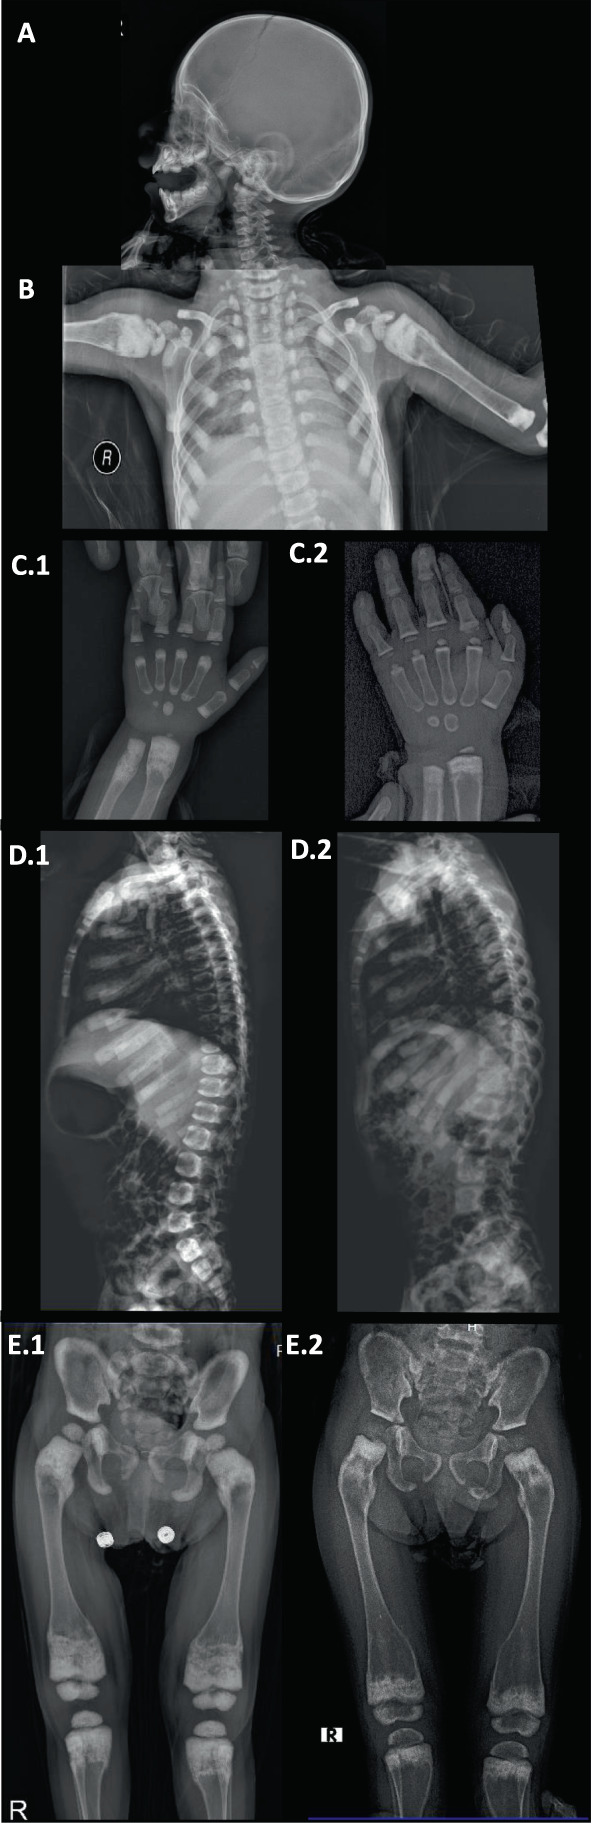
Patient 4. Radiographic findings in early childhood. (*A*) At age 2 years, the skull has slightly increased vertical diameter. (*B*) There is marked metadiaphyseal osteosclerosis and widening of the proximal humeri, marked osteosclerosis and some widening of the ribs, and osteosclerosis of the spine, clavicles, and scapulae. (*C.1*,*C.2*) Between ages 2‐1/3 and 4‐1/3 years, osteosclerosis has decreased in the metaphyses and carpals in the hands and wrists, and metadiaphyses in the radius and ulna. Metadiaphyseal widening has increased. (*D.1*,*D.2,E.1*,*E.2*) Osteosclerosis has decreased in the pelvis and lateral spine and metadiaphyses of the femurs and proximal tibias and fibulas. Metadiaphyseal expansion and cortical thinning are developing in the tubular bones.
